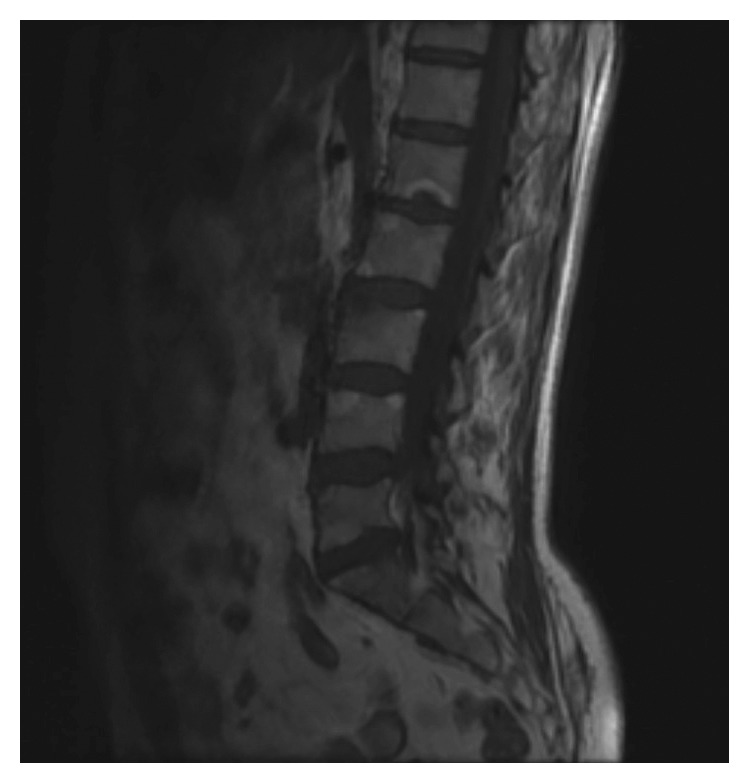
T1-weighted sagittal MRI scan of the lumbar spine indicating old Romanus lesions.
